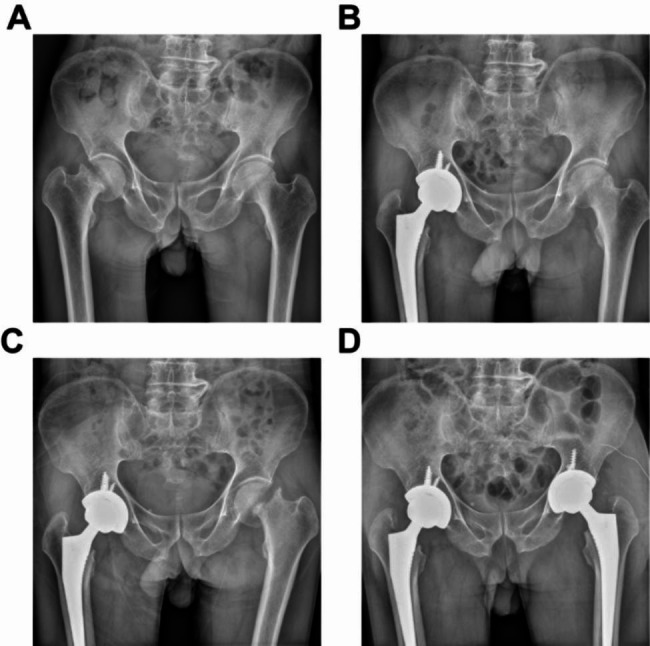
Anteroposterior radiographs of the patient’s hip joint before and after surgery
